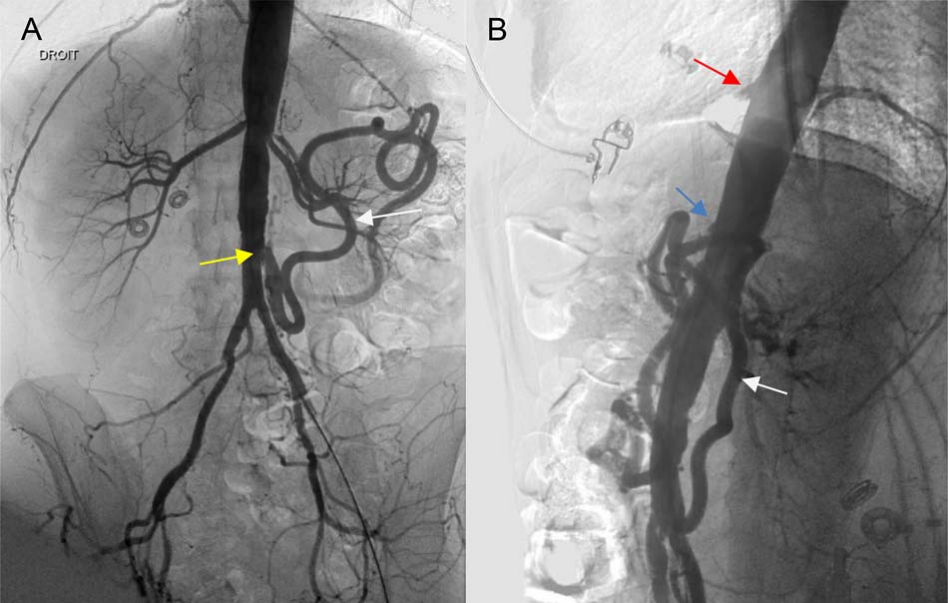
(A, B) Angiography of the abdominal aorta (preoperative), demonstrating the occlusion of the CT (red arrow) and SMA (blue arrow) with severe stenosis of the IMA (yellow arrow). Retrograde flow of the arc of the Riolan artery is shown (white arrow).
